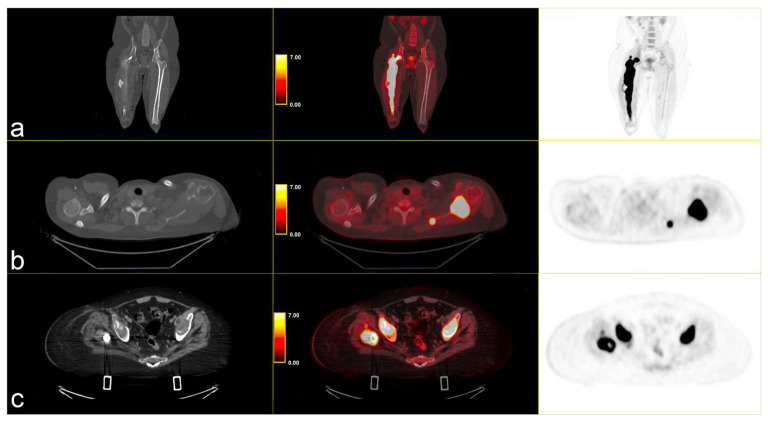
PET/CT fused images show large hypermetabolic lesions in (a) the right femur, (b) the left scapula (with erosion of the cortical part), (c) thickened osteogenic lesions in the pelvis and bilateral iliac wings (thickened osteogenic lesions in with lower SUVmax: 6.7). From left to right CT PET/CT fused images and PET images.
